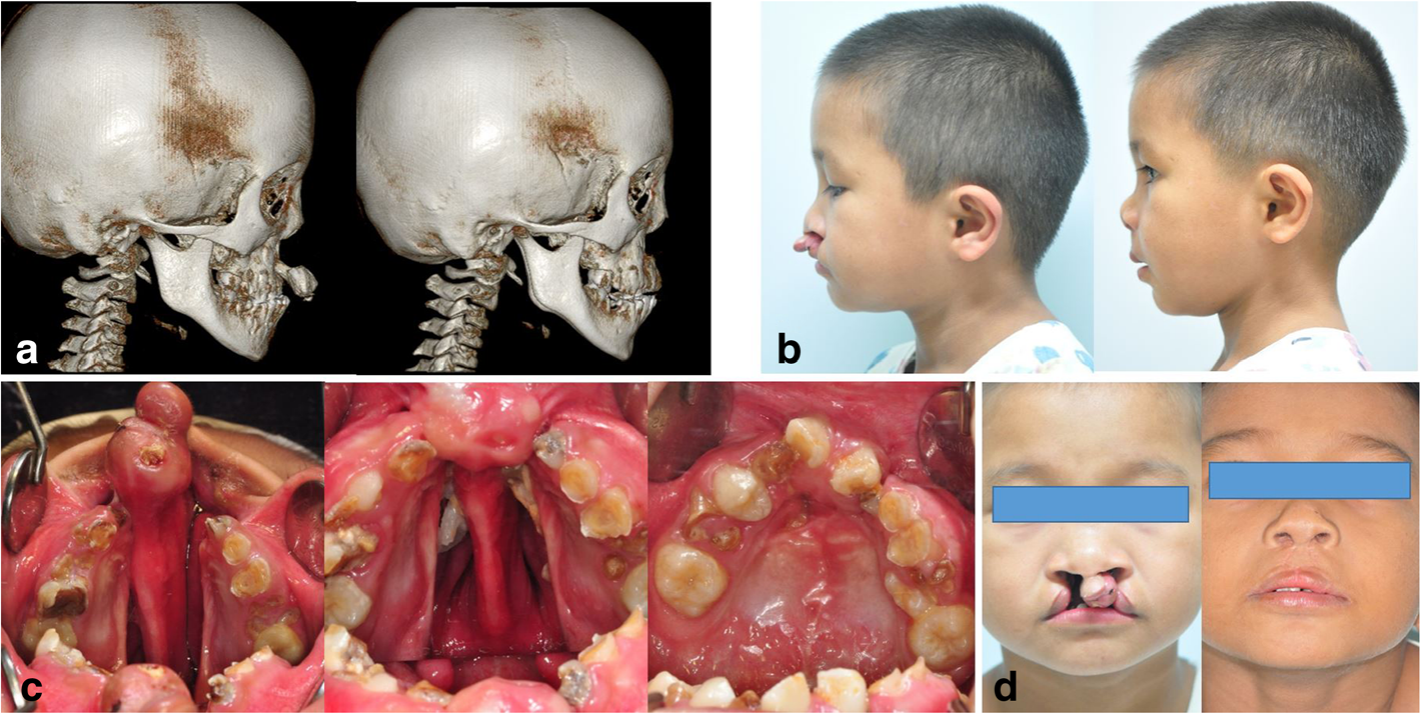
Case 3. a Preoperative and postoperative three-dimensional CT of an 8-year-old boy with a protruding premaxilla. b Preoperative and postoperative lateral photogram after concomitant premaxillary setback and nasolabial repair. c Mirror images of upper dental arch: preoperative, before palatoplasty, 2 years after the operation before alveolar bone grafting. d Preoperative and 2-year postoperative frontal photogram
